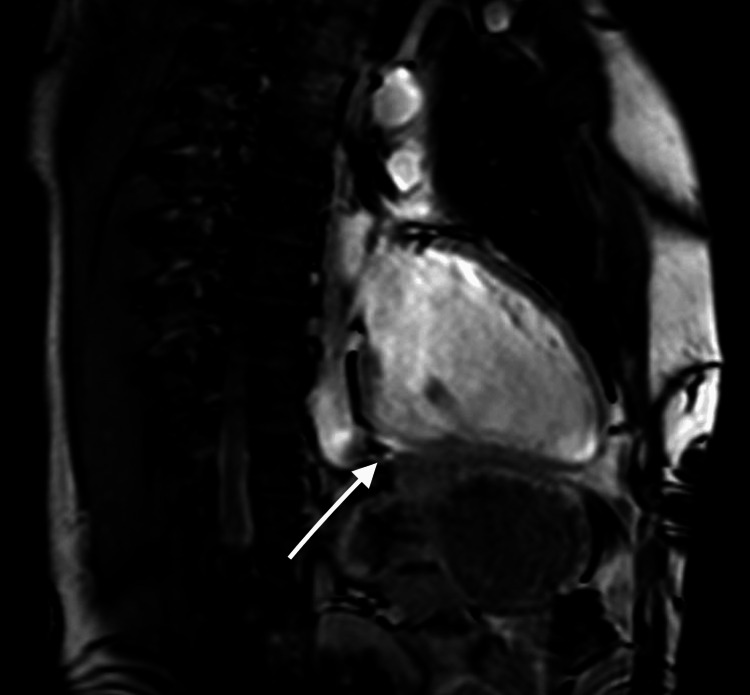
Cardiac MRI demonstrating a localized left ventricular aneurysm at the inferobasal wall. Sagittal cardiac MRI sequence showing a well-defined aneurysm of the inferobasal segment of the left ventricle. Late gadolinium enhancement indicates myocardial fibrosis consistent with a post-myocarditis sequela, which forms the substrate of the aneurysm.
